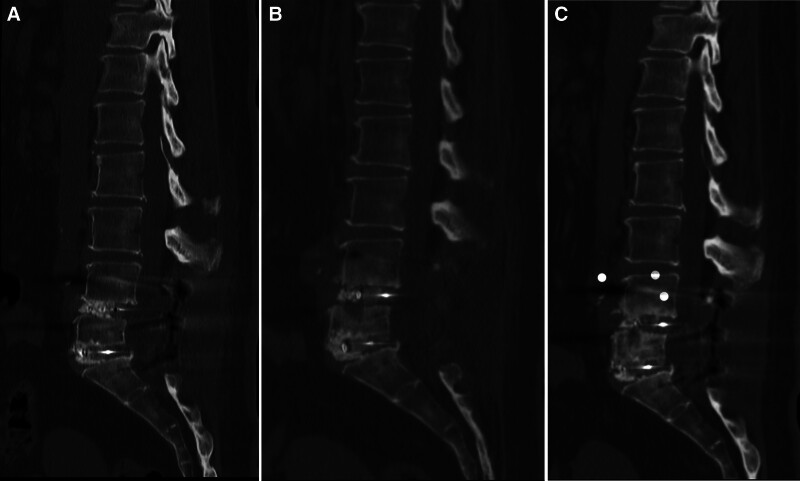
Sagittal CT imaging after surgery: (A) shows no evidence of ossification within the spinal canal on postoperative day 2. (B) shows no signs of ossification within the spinal canal on postoperative month 3. (C) continues to demonstrate no ossification within the spinal canal postoperative on month 6. CT = computed tomography.
